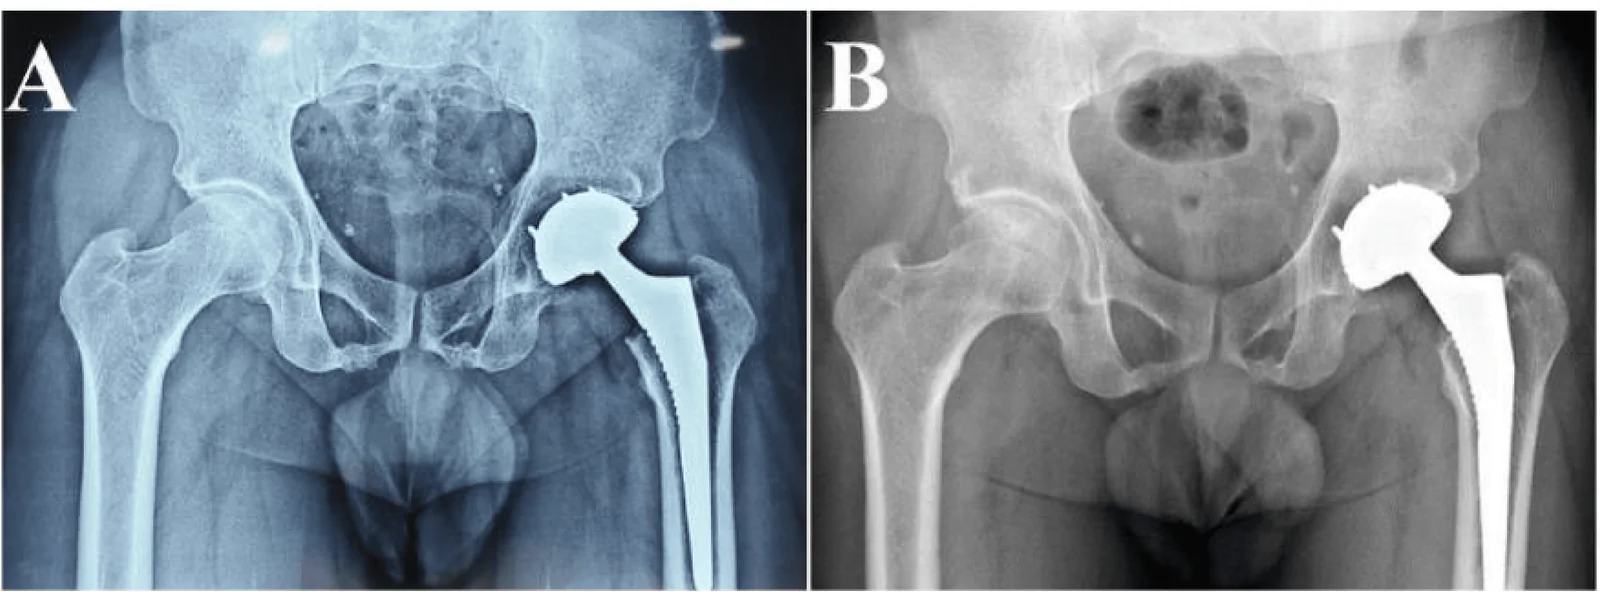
Preoperative and postoperative (24 months) X-ray of pelvis with hips (A) Preoperative X-ray of the pelvis showing the right hip with ONFH and the left hip with a total hip replacement prosthesis in situ; (B) 24-month follow-up X-ray showing sclerosis of the right femoral head indicative of osteogenesis, where core decompression with osteoblast cell therapy was done. ONFH: osteonecrosis of the femoral head
